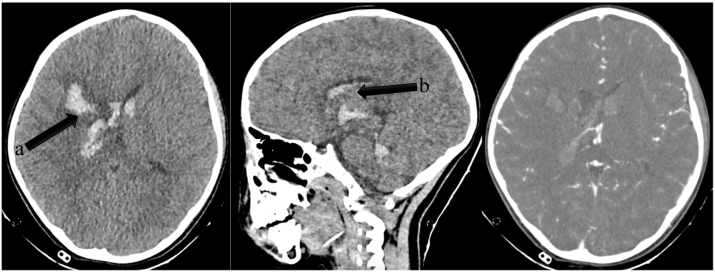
Head CT of the first case revealed a hyperdense lesion on the right frontoparietal lobe 4.6 × 2.7 × 4 cm in size and 24.8 cc in volume with an extension to the right lateral and third ventricle, and dilated ventricles, indicating the presence of right frontoparietal intracerebral (a) and intraventricular hematoma (b) along with non-communicating hydrocephalus. CT angiography (CTA) was performed but did not reveal any apparent vascular malformation.
